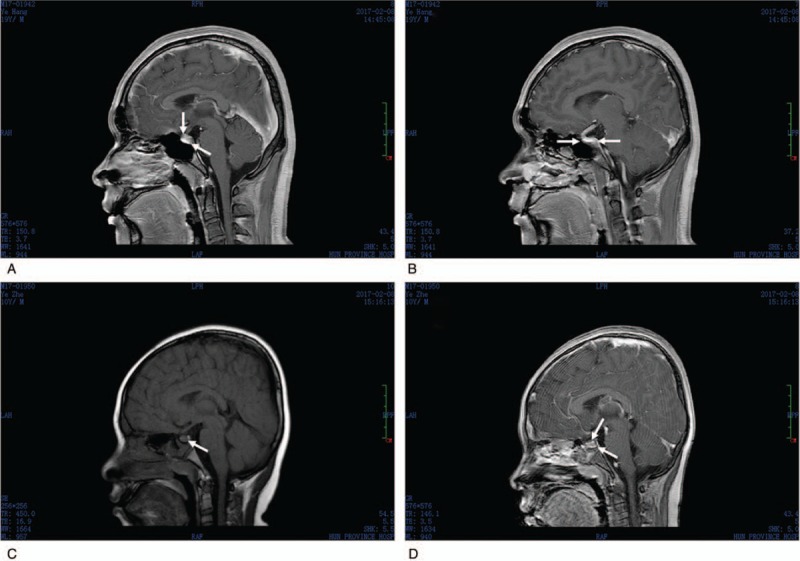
The MRI results from the patients. Patient 1 with cysts at sinusoid (A, B); Patient 2 showed a large amount of fluid stored within the sinus cavity, resulting in sinusitis (C, D). MRI = magnetic resonance imaging.
